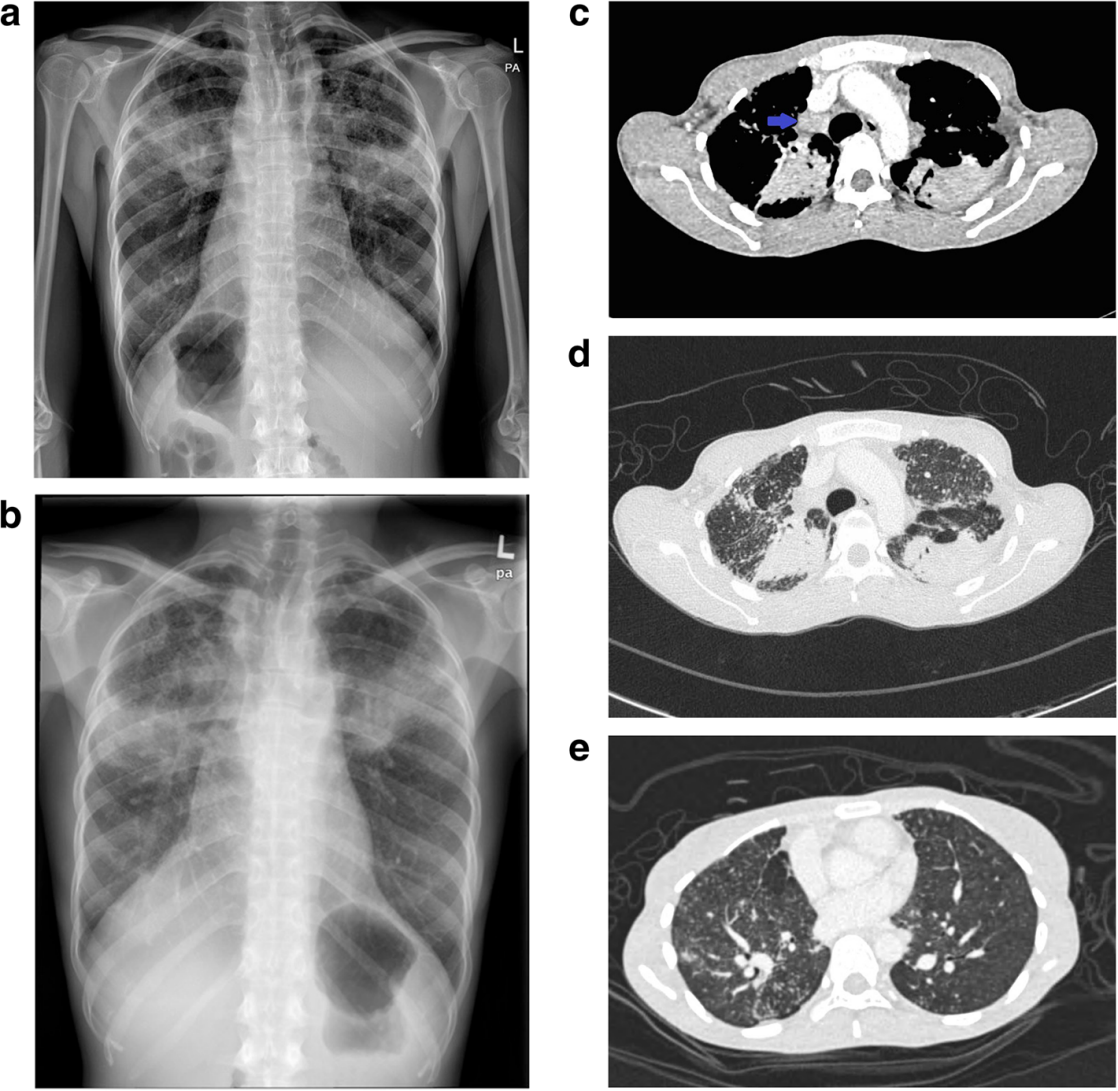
a Chest x-ray showed fibrotic changes at both upper zones with reticulo-nodular opacity over the upper and middle zone of both lungs. b Repeated chest x-ray after 54 doses of anti tuberculous medications showed absence of radiological improvement with similar reticulo-nodular opacity over bilateral lung field. c Mediastinal view of CT thorax showed enlarged right paratrachea lymphadenopathy (*Blue arrow*). d Consolidative changes with air bronchogram at the posterior segment of the left upper lobe and the apico-posterior segment of the right upper lobe. e The presence of lung nodules with tree in bud appearance
